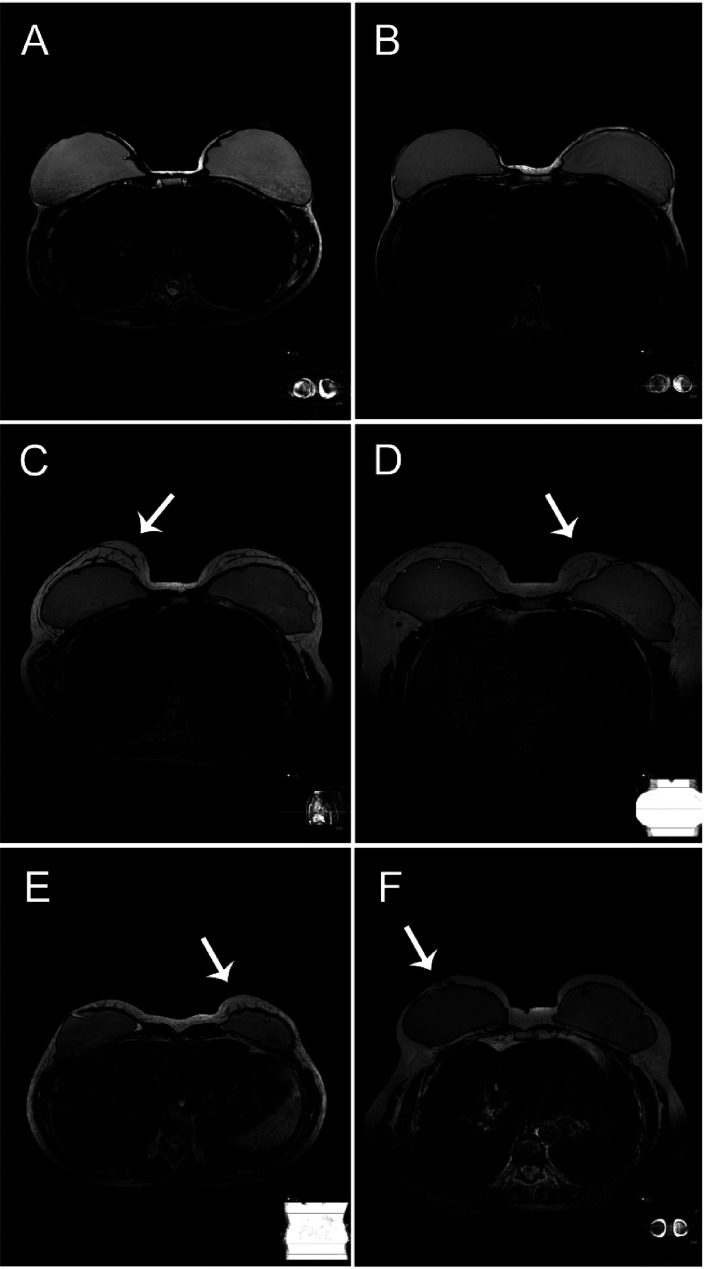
Examples on T2-weighted MRI images on women with and without residual glandular tissue postoperatively: (a, b) women with thin skin flaps without detectable residual glandular tissue; (c) woman with thick skin flaps with agreement on presence of residual glandular tissue; (d, e) women with possible residual glandular tissue where the observers disagreed whether it was residual glandular tissue, ligaments, scar tissue, artefacts, or other; (g) woman operated with nipple areolar-conserving technique with residual glandular tissue located in a retromammary area. Areas with suspected residual glandular tissue are highlighted with a white arrow. MRI, magnetic resonance imaging.
